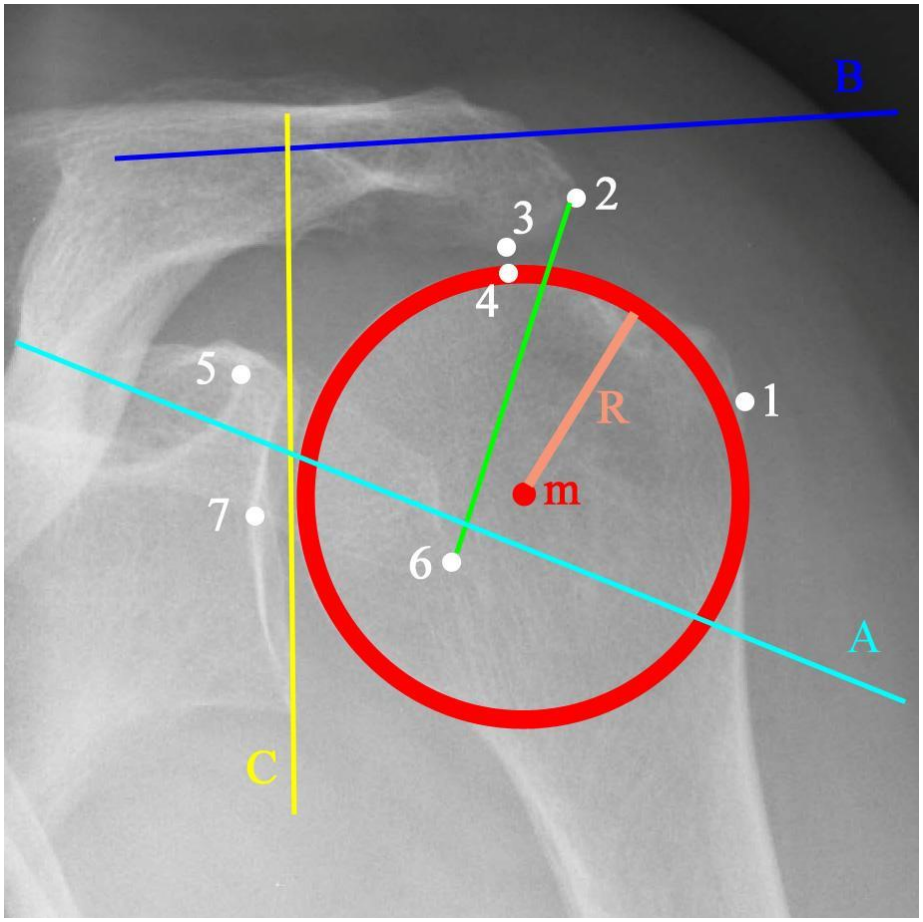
Example of the marking points and lines drawn onto each radiograph.
